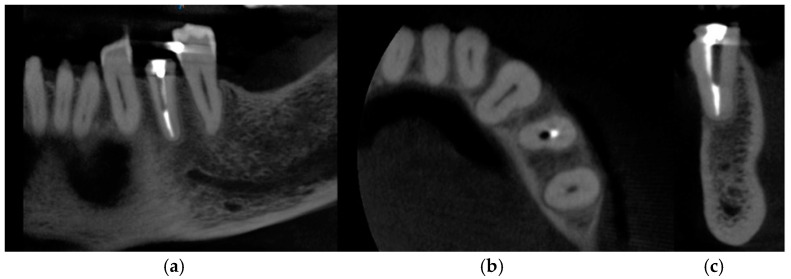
CBCT images of the patient before the surgery: (a) frontal section, (b) transversal section, and (c) sagittal section.
